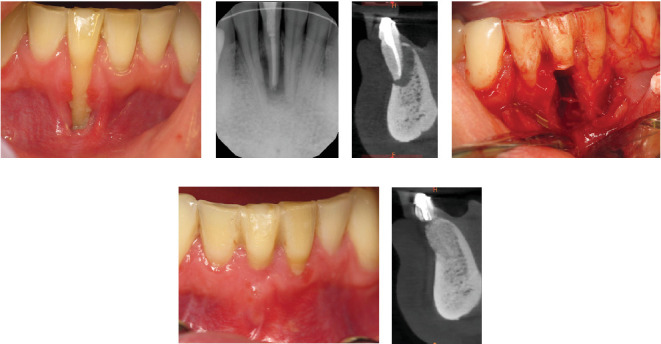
Mandibular incisor with mucogingival defect (A) and periapical periodontitis (B and C) before extraction. Tooth was extracted (D) and crown used as a pontic in provisional prosthesis and splinted to the adjacent teeth. Favorable healing was observed clinically (E) and radiographically at a 2-year follow-up (F).
